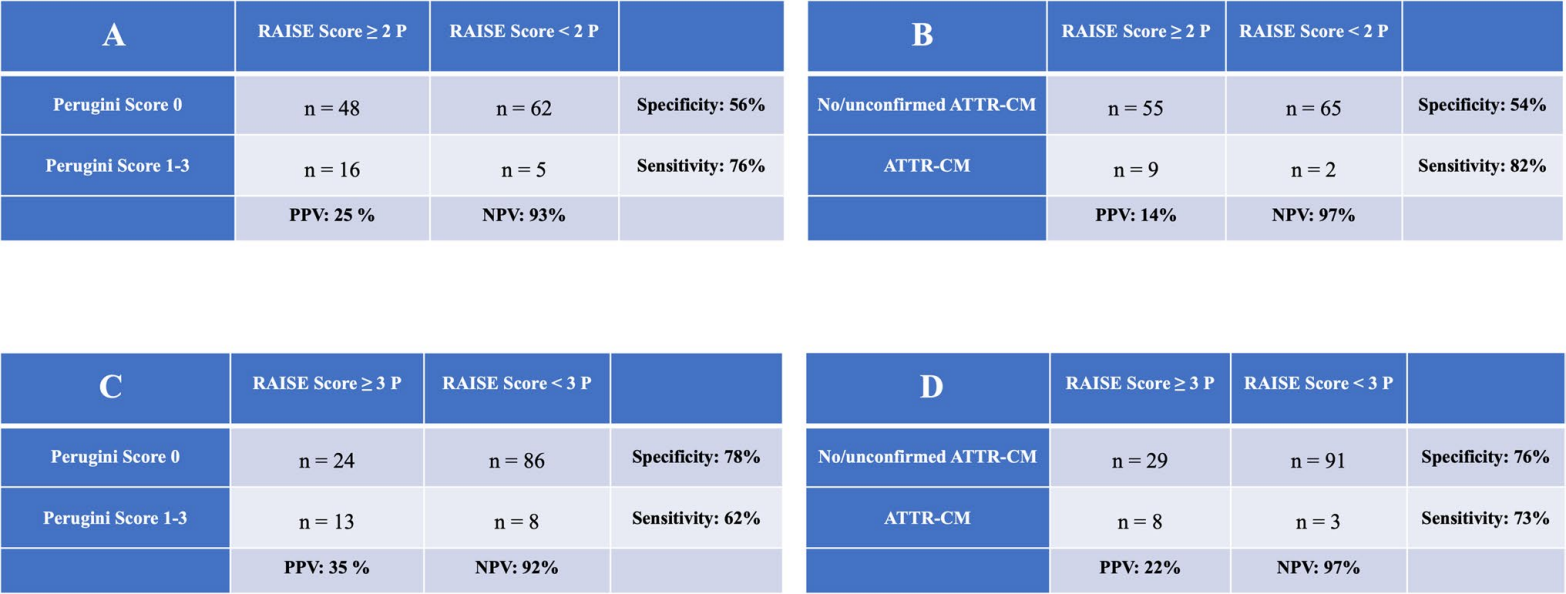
Sensitivity, specificity, PPV, and NPV for the RAISE Score with cutoff values of 2 (A and B) and 3 (C and D) regarding pathological bone scintigraphy result and definitive ATTR-CM diagnosis
